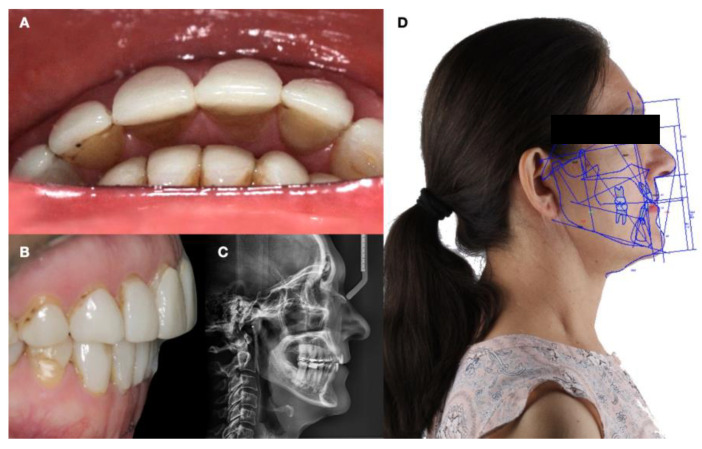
Initial situation. (A) Overjet evaluation incisal view; (B) overjet evaluation lateral view; (C) cephalometric radiograph; and (D) orthodontic analysis based on cephalometry.
